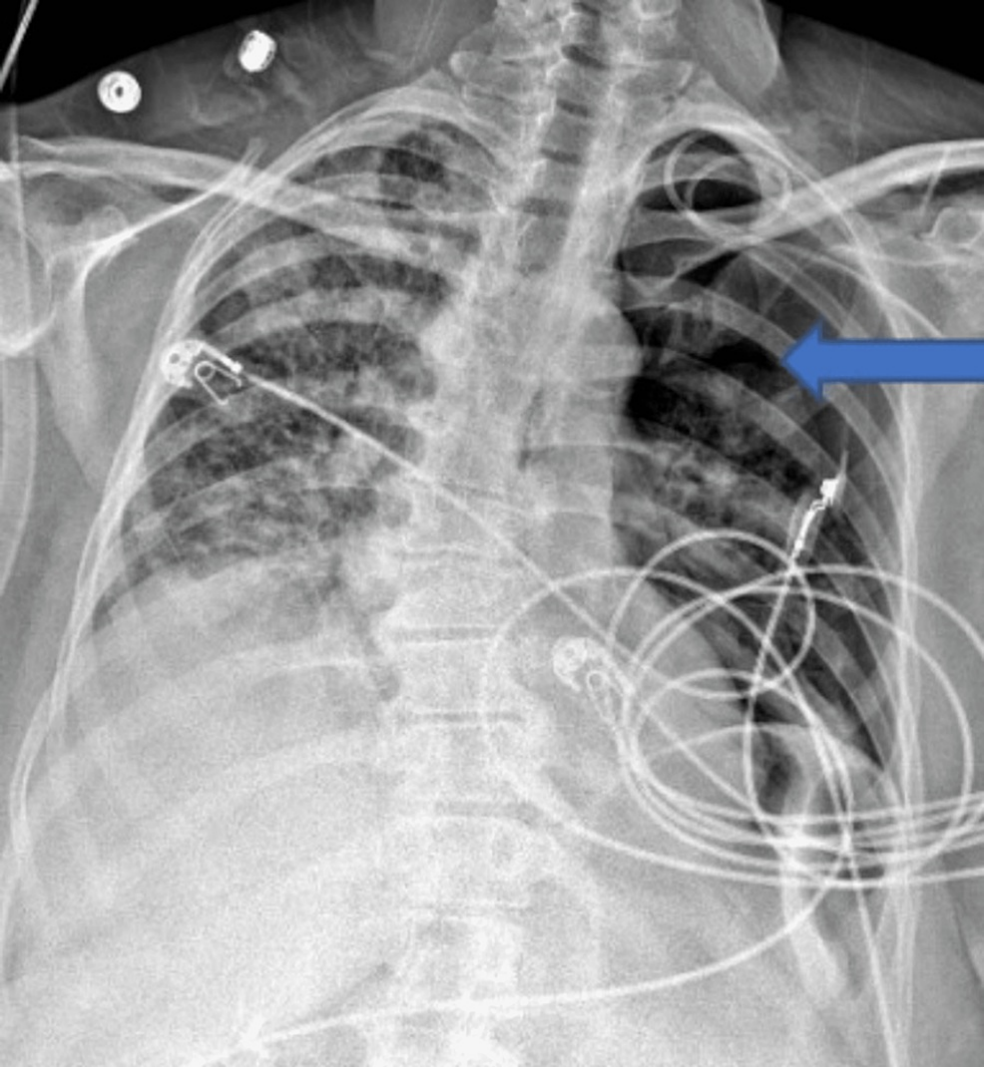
Chest X-ray showing increasing left-sided pneumothorax (blue arrow)
